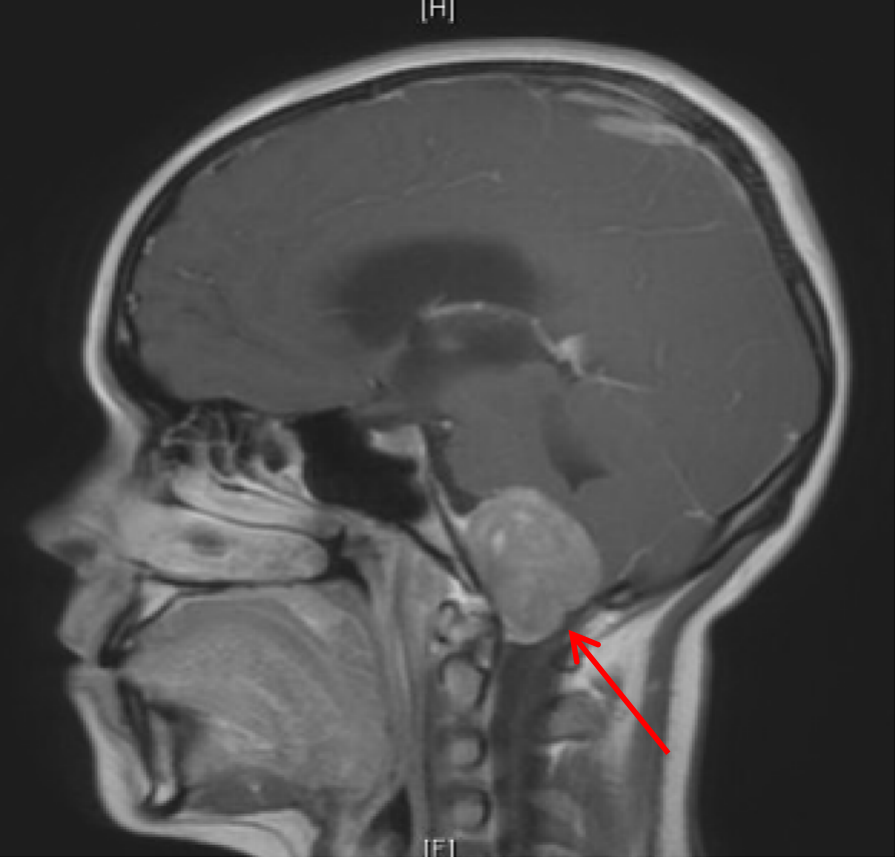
MRI head of a 17-year-old female who developed a basal skull Ewing sarcoma 5.3 years after starting etanercept therapy
